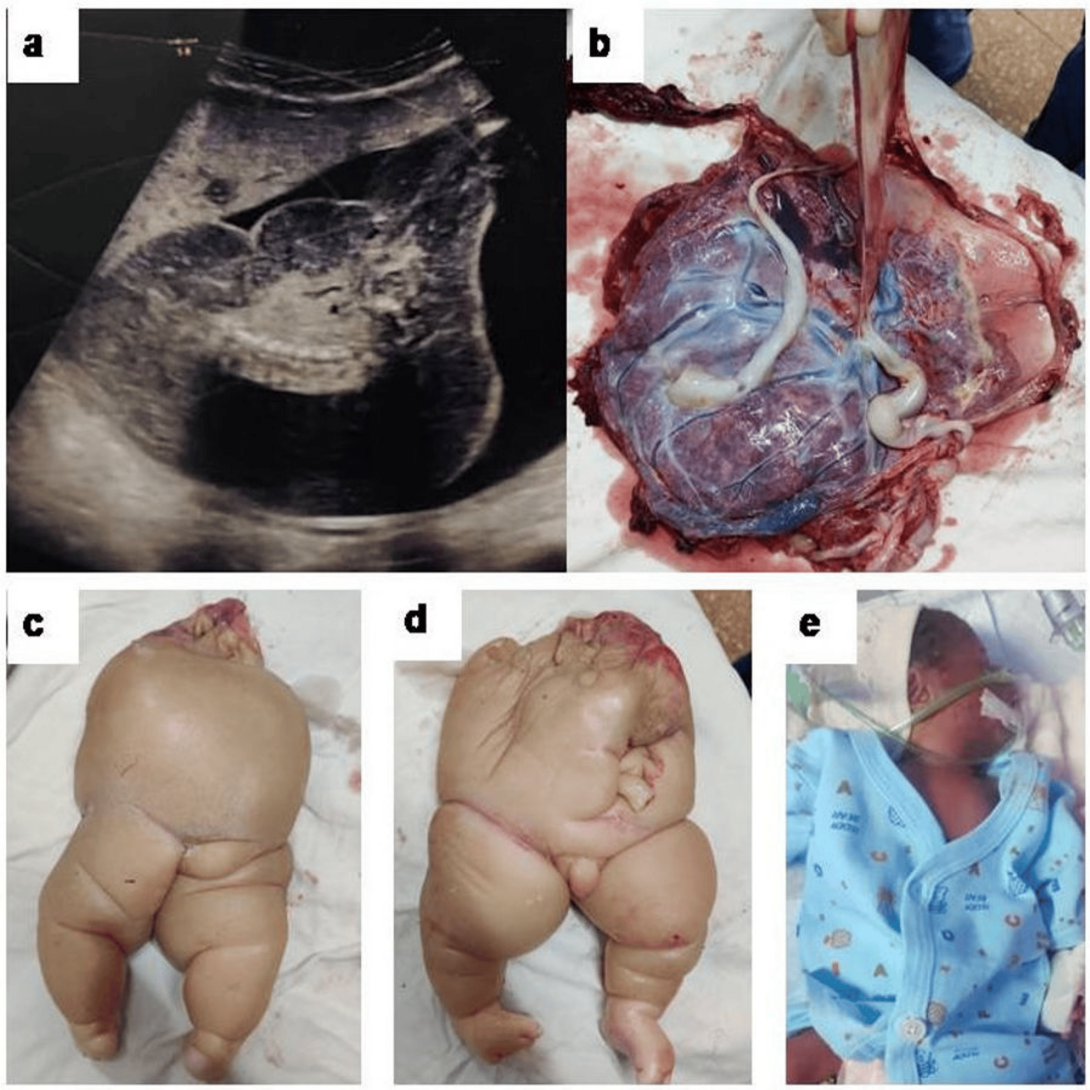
Ultrasonographic and gross images of the acardiac twin and pump twin a) Ultrasound picture of acardiac twin seen as a large heterogenous intrauterine mass with spine-like calcification b) Monochorionic diamniotic placenta c-d) Acardiac twin e) Pump twin
